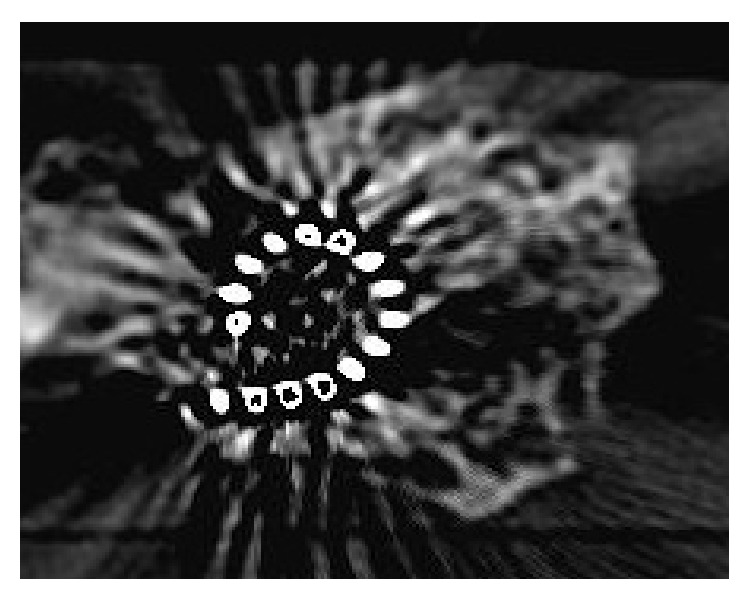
Computed tomography imaging with visualization of the electrode array within the cochlea.
